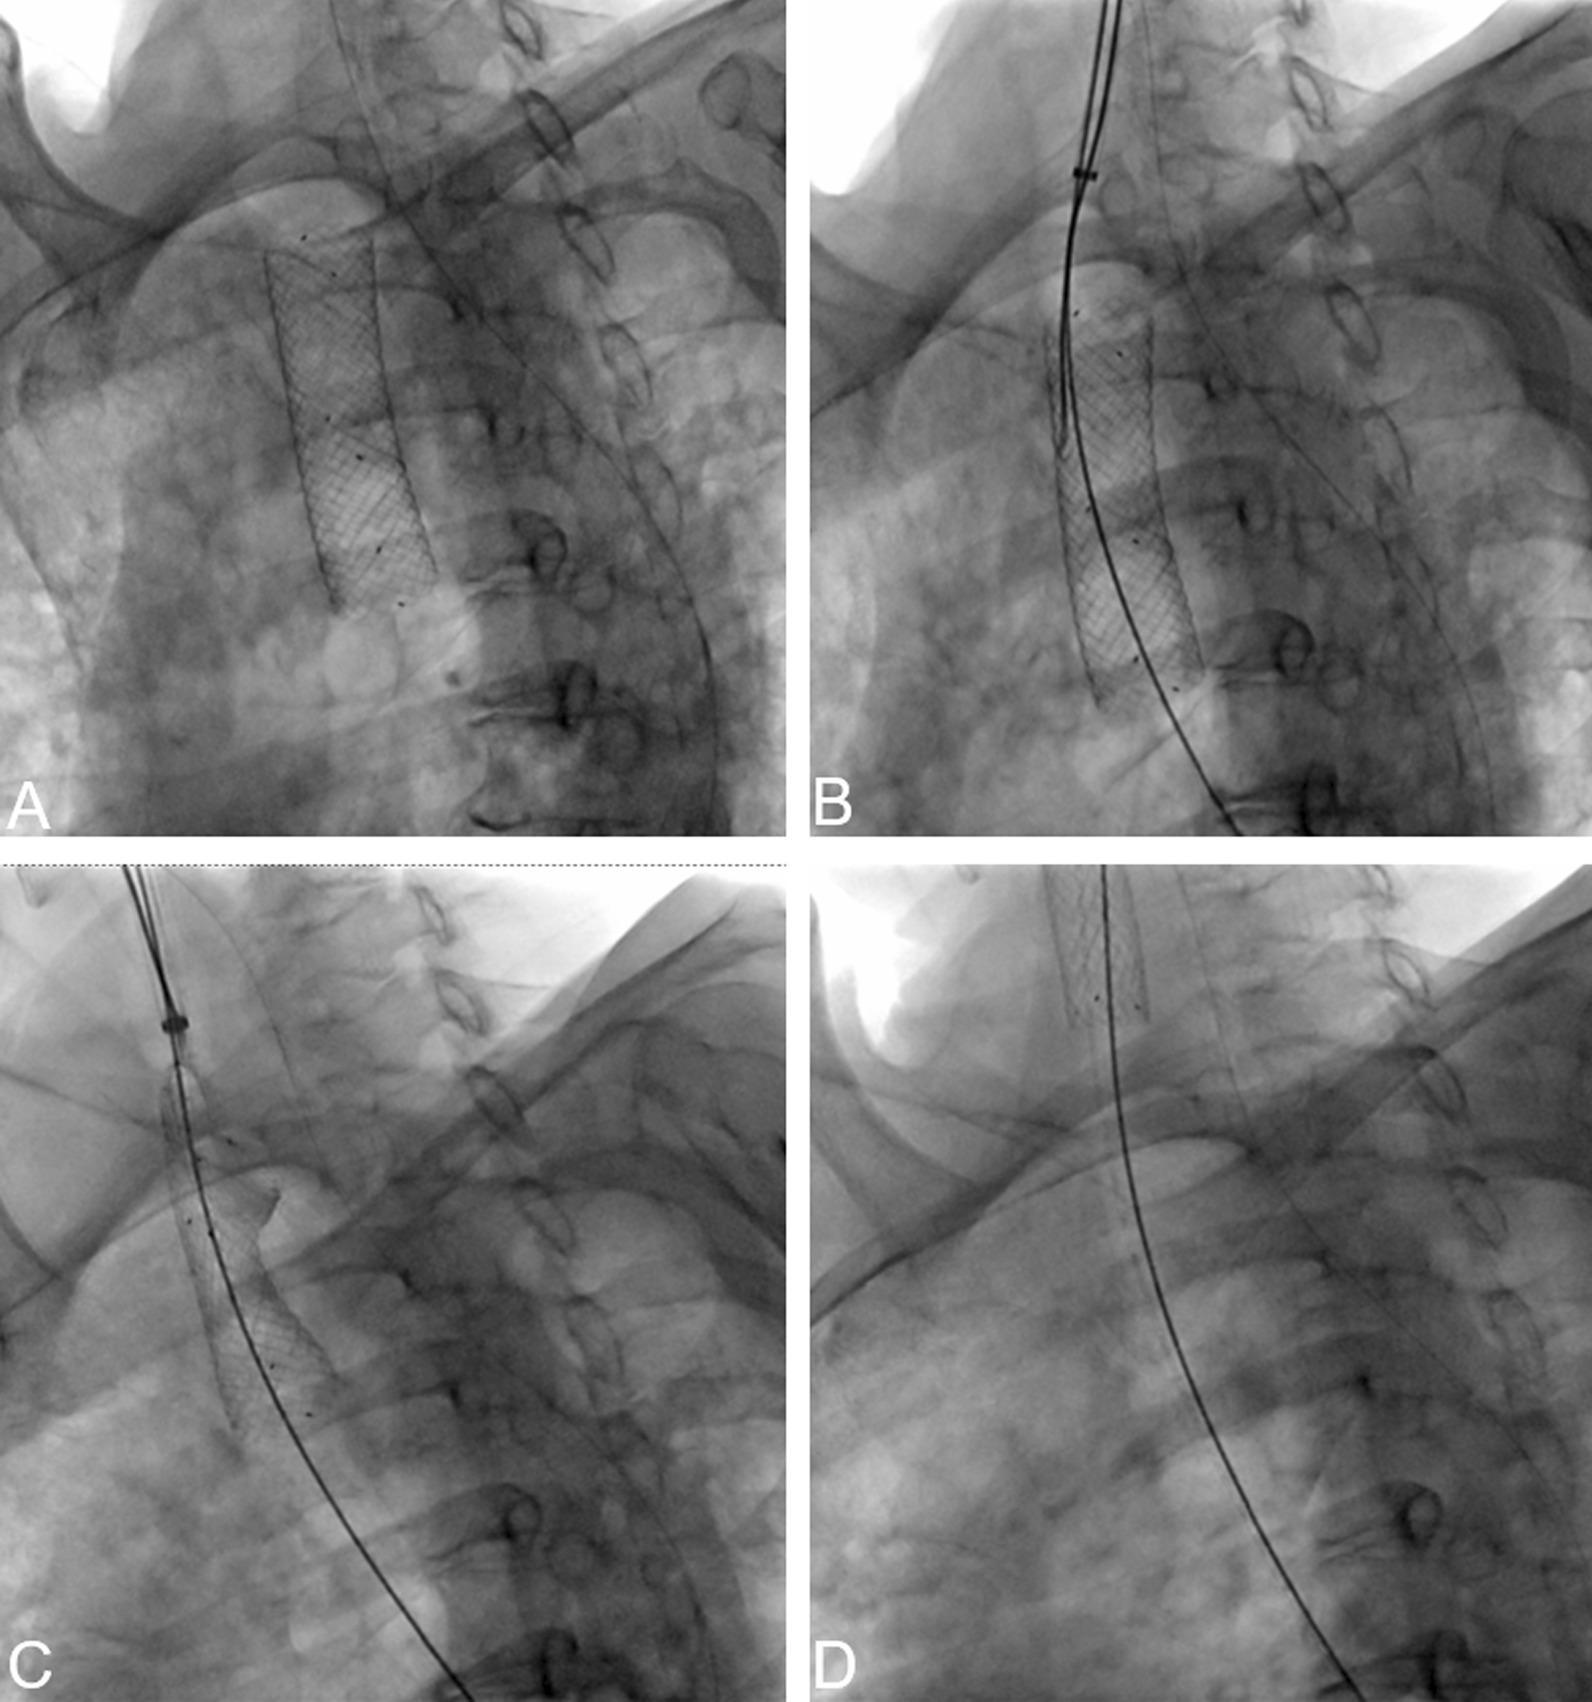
A 62-year-old female with tracheal stenosis caused by postoperative recurrence of esophageal cancer underwent tracheal covered stent implantation for more than 2 months. The patient subsequently experienced dyspnea for 7 days. CT showed that the tracheal stent had moved downward. DSA (A) showed that the tracheal stent had shifted downward. DSA (B) showed that stent-removal hook hooked the upper end of the tracheal stent. DSA (C–D) showed that the tracheal stent was then removed by direct extraction method, in which the stent-removal hook was attached to the upper end of the tracheal stent to collapse the proximal end. The patient’s dyspnea was relieved after the stent was removed
